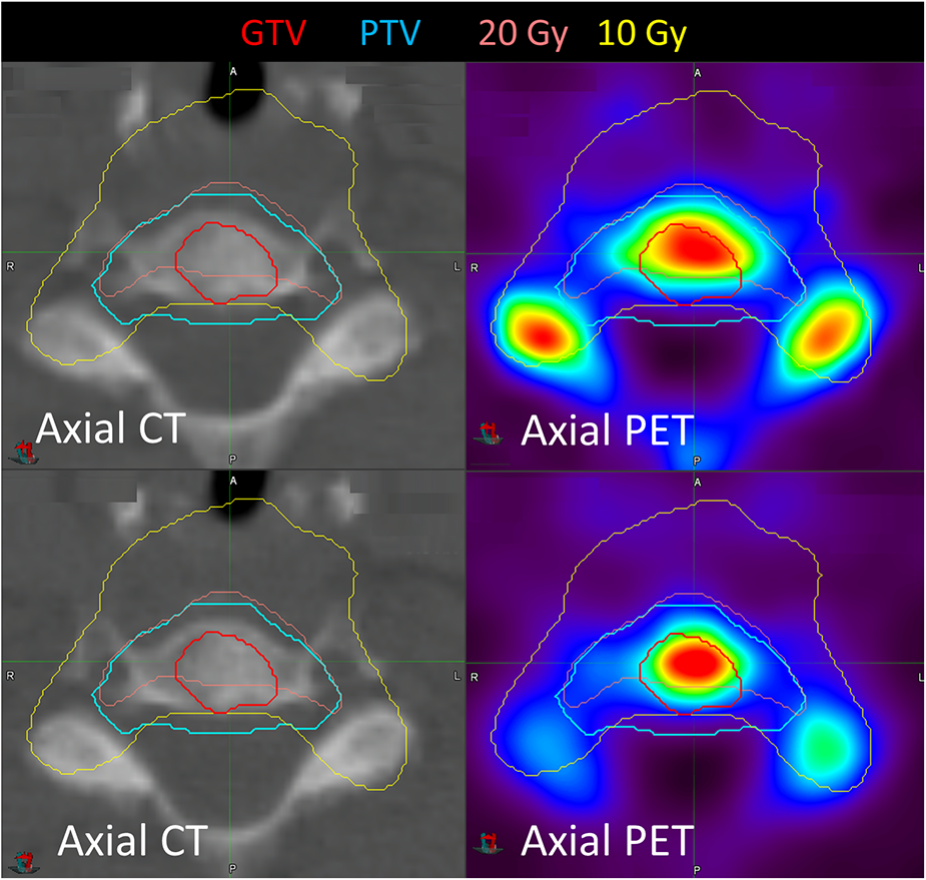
Pre- and post-treatment imaging for patient 14 who had a C6 metastasis treated. SUV_max_ increased after treatment from 11.0 to 12.0, but SUV_mean_ decreased from 7.0 to 6.3.
